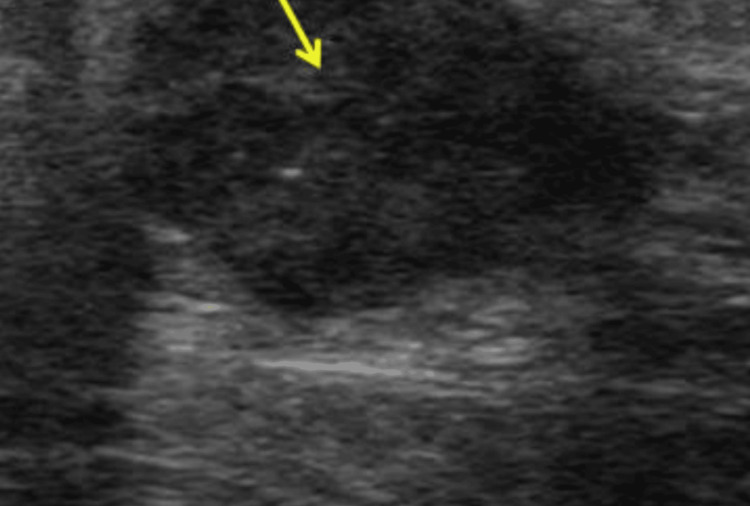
Initial ultrasound of left breast findings Ill-defined hypoechoic lesion (yellow arrow) with radiating borders and adjacent ductal dilatation reaching upto the nipple of size ~2.8x2 cm in 3 o’clock position within zone 2b-BIRADS 4.
